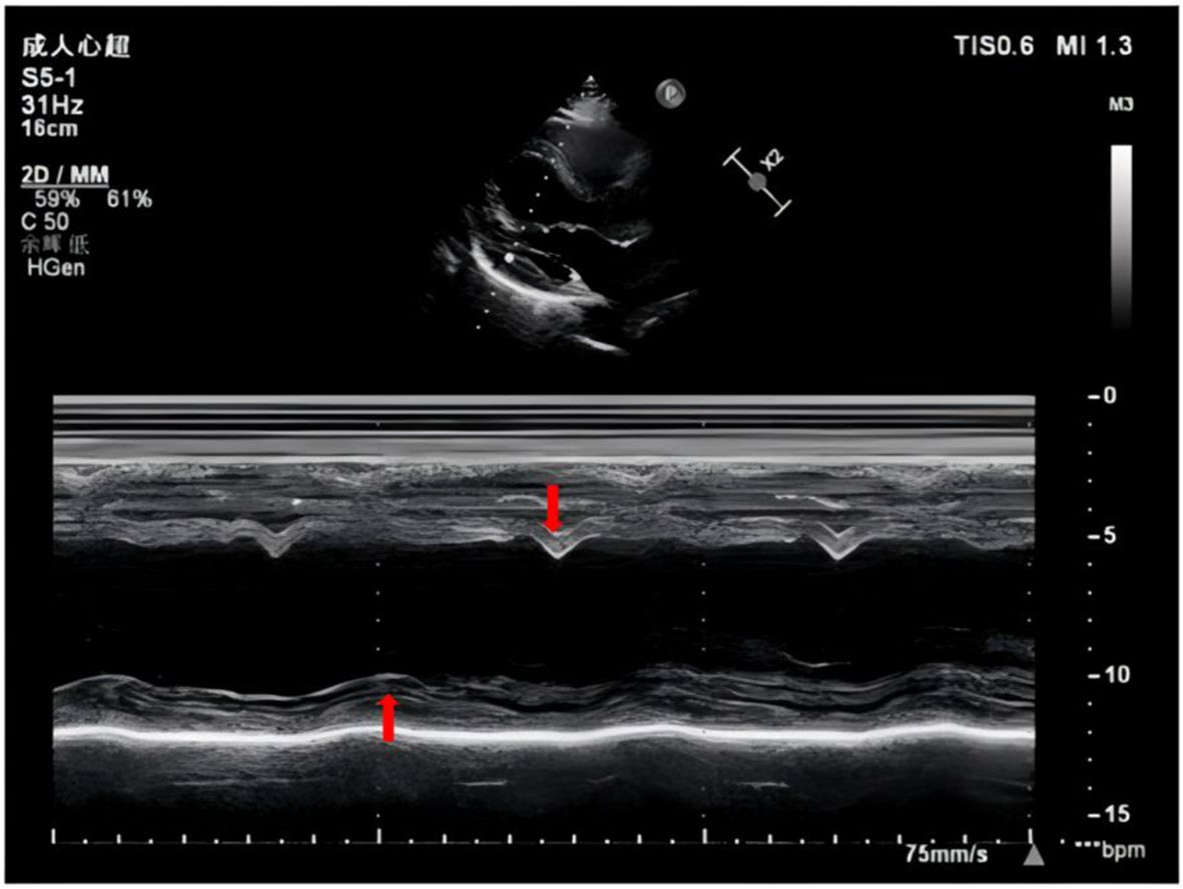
M-mode echocardiography showing inter-ventricular septal–posterior wall motion asynchrony.M-mode echocardiography showing septal–posterior wall motion asynchrony; the vertical bars mark the time difference between peak inward motion of the inter-ventricular septum (IVS) and the posterior wall (PW)
